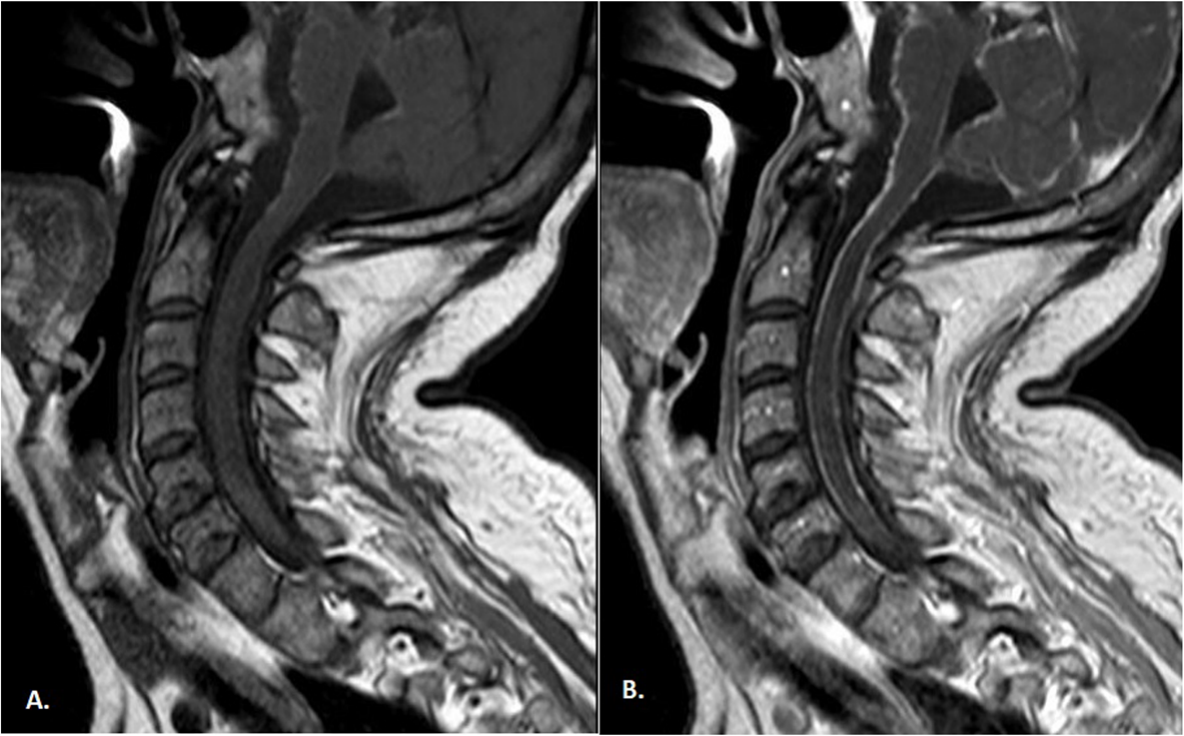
Cervical spinal cord MRI T1-weighted imaging without (a) and with (b) gadolinium contrast enhancement. Hyper-intensity on T1 was due to the paramagnetic effect of melanin, which had stable organic free radicals inside it, resulting in shortened T1 relaxation times in typical melanotic melanoma
